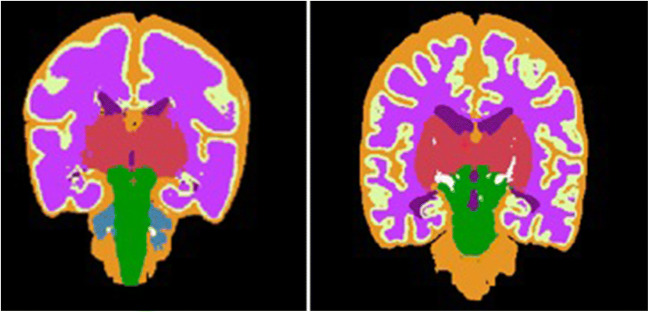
Examples of an automatically segmented MRI at a 30 weeks PMA and b 40 weeks PMA. Volumes are indicated according to the following color scheme: red: deep grey matter; pink: unmyelinated white matter; purple: ventricles; yellow: cortical grey matter; orange: cerebral spinal fluid; white: myelinated white matter; green: brain stem. blue: cerebellum
